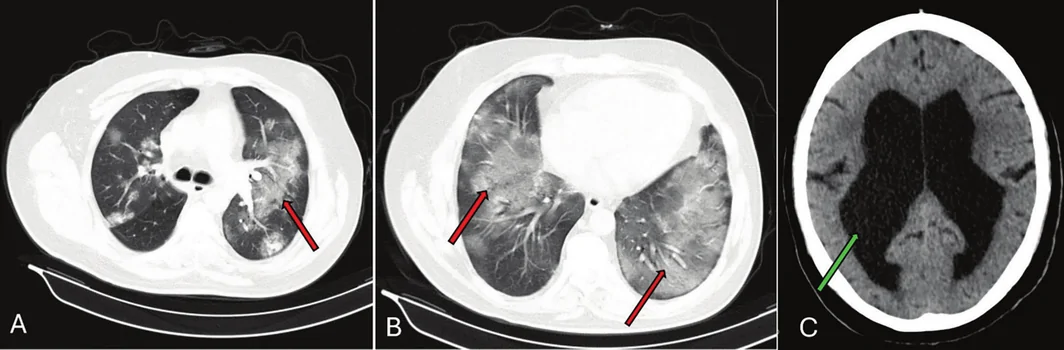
Ground‐glass opacities and patchy consolidation throughout the parenchyma of both lungs, which indicate DAH in the lung CT scan (A&B, red arrows). Ventriculomegaly and hydrocephalus in the brain CT scan (C, green arrow).
